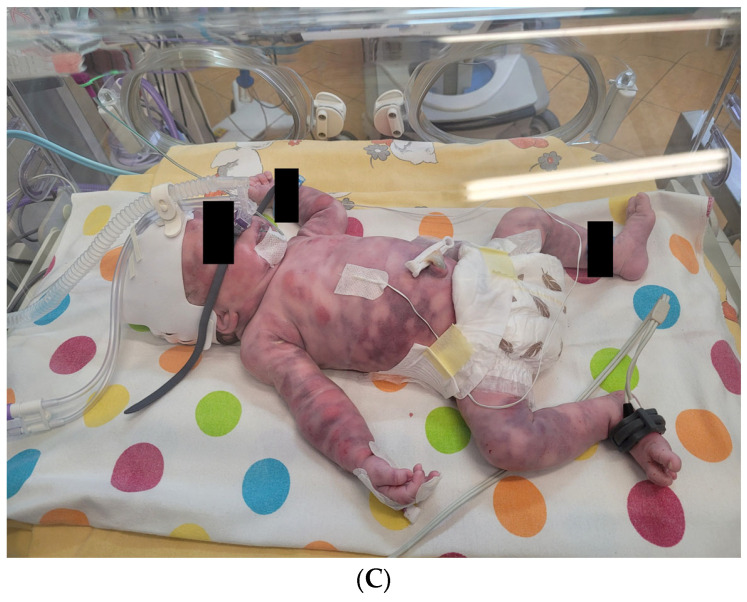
(A) Ultrasound image presenting amniotic fluid index measurement. (B) Ultrasound image presenting foetal abdomen and tumour in the hepatic hilum area. (C) Bruisings and nodular lesions on the skin of the neonate with congenital neonatal leukaemia.
